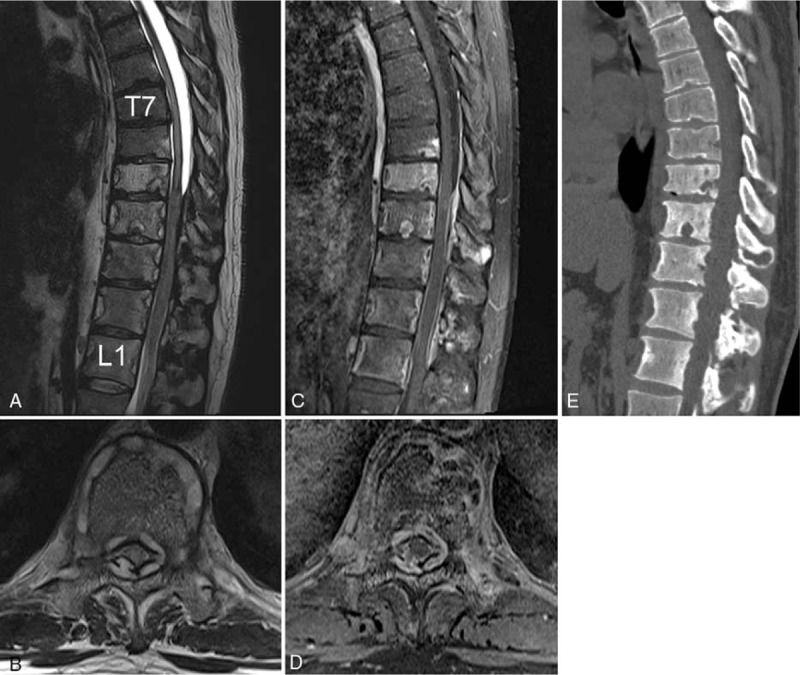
Preoperative T2-weighted (A and B), T1 gadolinium-enhanced (C and D) sagittal MR images, and axial images at the level of T10, (E) a sagittal CT reconstructive image showing a homogeneous continuous enhancing epidural lesion and osteolytic bone lesion at the T7–L1 level.
